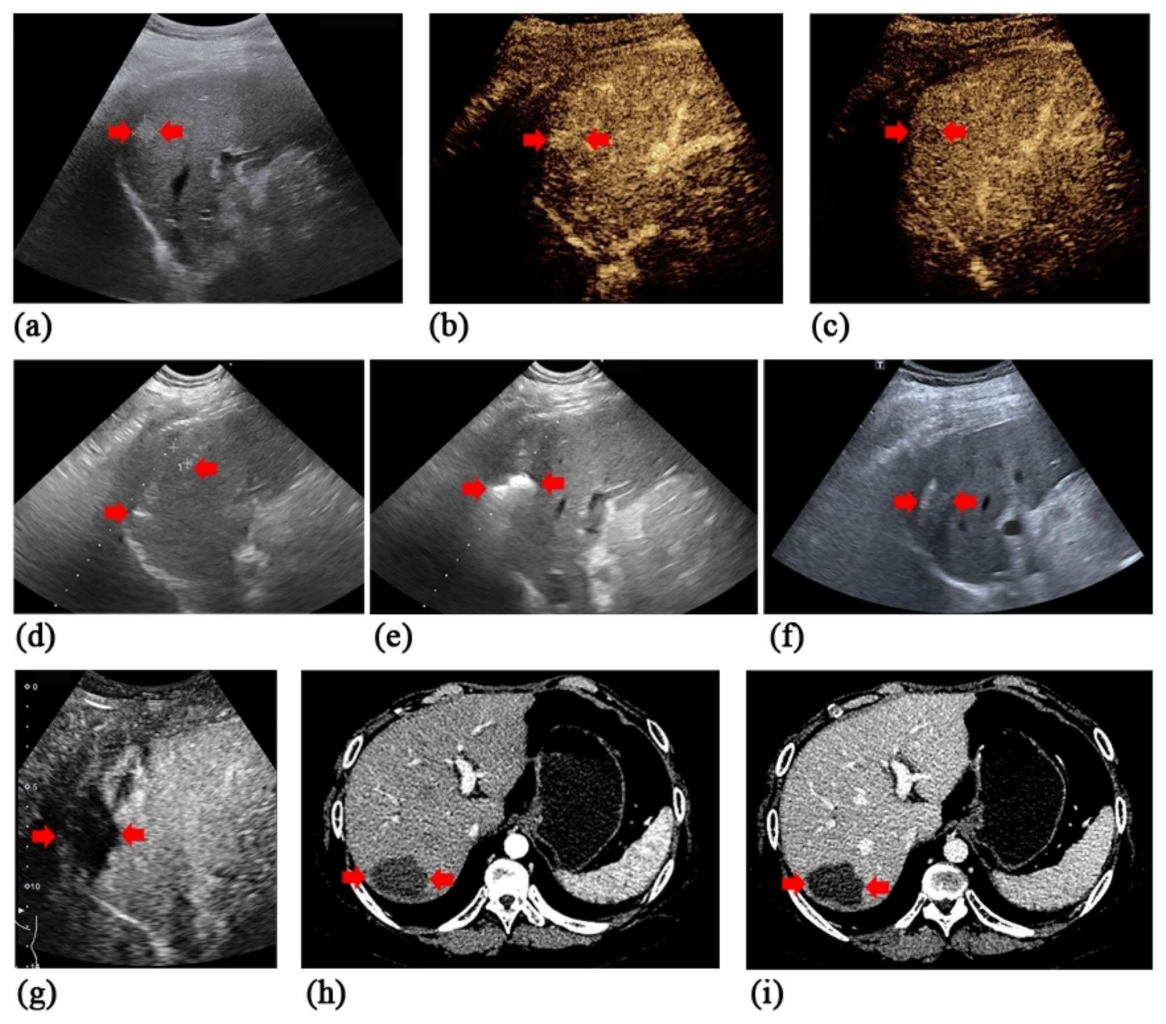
A 14-year-old male was diagnosed as intrahepatic recurrent hepatocellular carcinoma (RHCC) at 7 months after initial liver transplantation (LT). Ultrasound-guided radiofrequency ablation (RFA) was performed. (a) A hyperechoic lesion located in Segment 7, with a size of 1.4cmx1.2cmx1.2cm was detected by the grayscale ultrasound. (b, c) On the contrast-enhanced ultrasound (CEUS) images, the targeted lesion displayed homogeneous hyperenhancement in the arterial phase (b) and washout in the portal phase (c). (d) Under the guidance of real-time ultrasound, the No-touch technique was performed by sequentially inserting two electrodes around the periphery of the target lesion and activating them alternatively to perform ablation with a sufficient peri-tumoural margin and avoid direct puncture of the tumor. The second radiofrequency electrode was being inserted, with an inter-electrode distance of 1.1 cm. (e) Hyperechoic zone around the electrode tips appeared and the range extended as the RFA procedure proceeded. (f, g) The next day after RFA, the ablative zone exhibited as heterogeneous hyperechoic on the grayscale ultrasound (f) and displayed no enhancement in all phases on the CEUS images, indicating a complete ablation (g). (h, i) Two months after RFA, the target lesion exhibited non-enhancement on the contrast-enhanced CT (CECT) images
